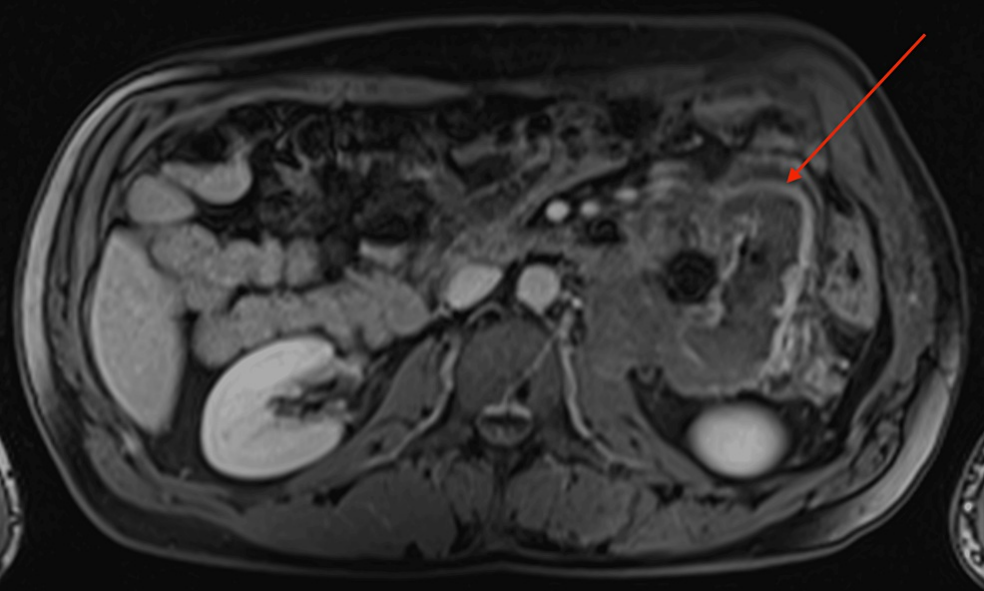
Axial MRI T1-weighted image, with intravenous contrast infusion Note the heterogeneity, the infiltration of the left psoas muscle, and the peripheral signal enhancement after intravenous contrast infusion MRI: magnetic resonance imaging
